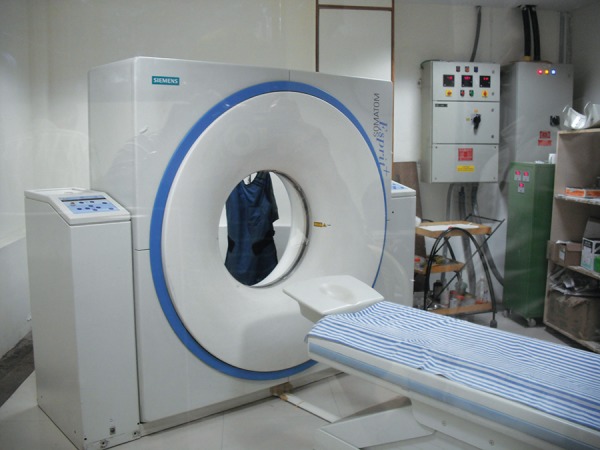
Spiral computed tomography unit (Siemens® SOMATOM Esprit + CT)
